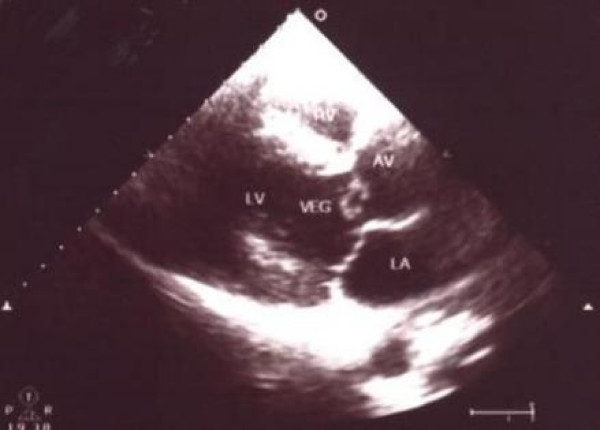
Short axis view on transesophageal echocardiography with valve thickening and an attached echogenic mass (vegetation).
